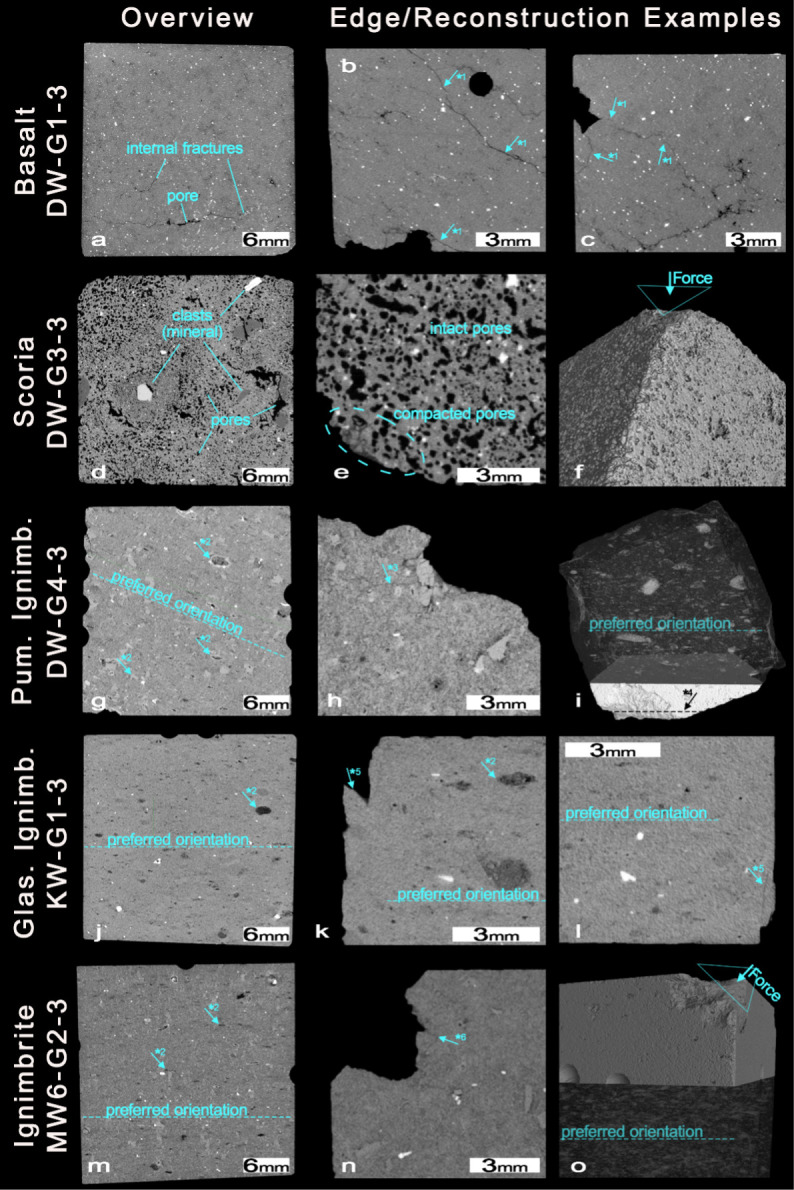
CT data of the used cubes with overviews as centred cross section in the 1^st^ column and close-up views on the cube’s impact corners in the 2^nd^ and 3^rd^ columns. a. The basalt cube with pores connected to the internal fracture network. b-c. Close-up view of impact edges with breaking along or parallel to internal fractures (*1). d. The scoria cube characterized by a high abundance of pores and clasts of various lithology. e. Compacted pores off an impact corner. f. Reconstruction of impact corner showing its parallel surface to the impact surface. g. The pumaceous ignimbrite cube with a medium-strong preferred orientation formed by clasts and pores (*2). Partly, the pores show rims of remaining mineral h. Close-up view on impact corner with large material loss and induced impact fractures (*3). i. Reconstruction of the pumaceous ignimbrite cube with the upper part showing the preferred orientation of high CT-number clasts and the lower part showing the breaking edge of an impact corner which is parallel to the preferred orientation (*4). j. The glassy ignimbrite with a weak preferred orientation by pores and clasts (*2). k-l. Close-up view on impact corners with small scale chipping off at breaking edges in high angle to the preferred orientation (*5). m. The “regular” ignimbrite with medium-strong preferred orientation of pores and clasts. n. Close-up view on impact corner with large material loss and induced impact fractures (*6). o. Reconstruction of an impact edge with the impact surface not affected by the preferred orientation inside the cube shown in the lower half of the cube.
